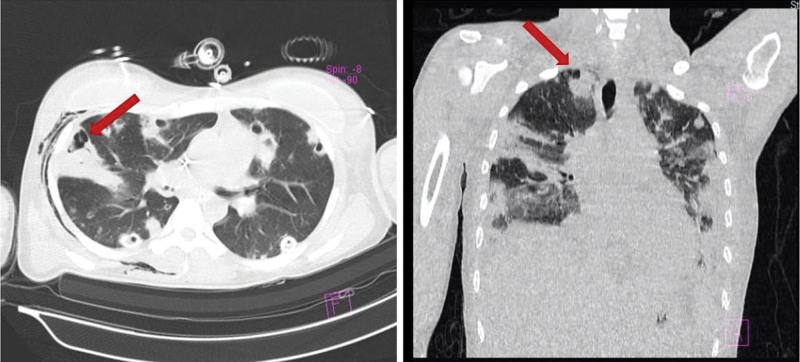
On day 5th of hospitalization, a contrast thoracic CT scan showed multiple necrotic areas with confluent cavities in both lungs (red arrows) and massive pleural effusion.
